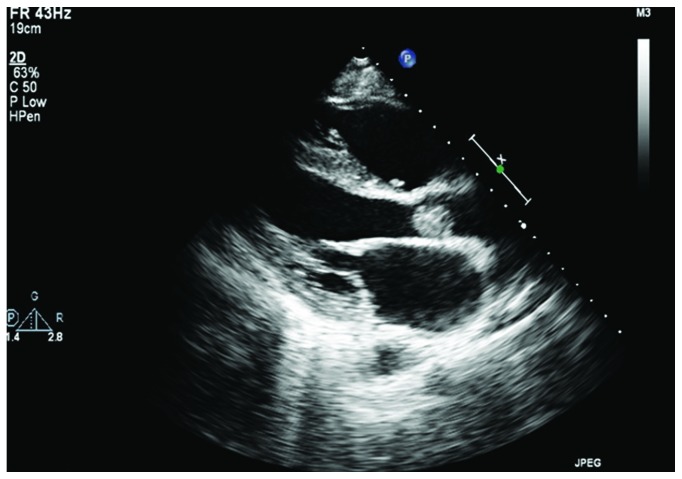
Echocardiogram demonstrating a vegetation measuring 1.8 cm × 1.3 cm.
